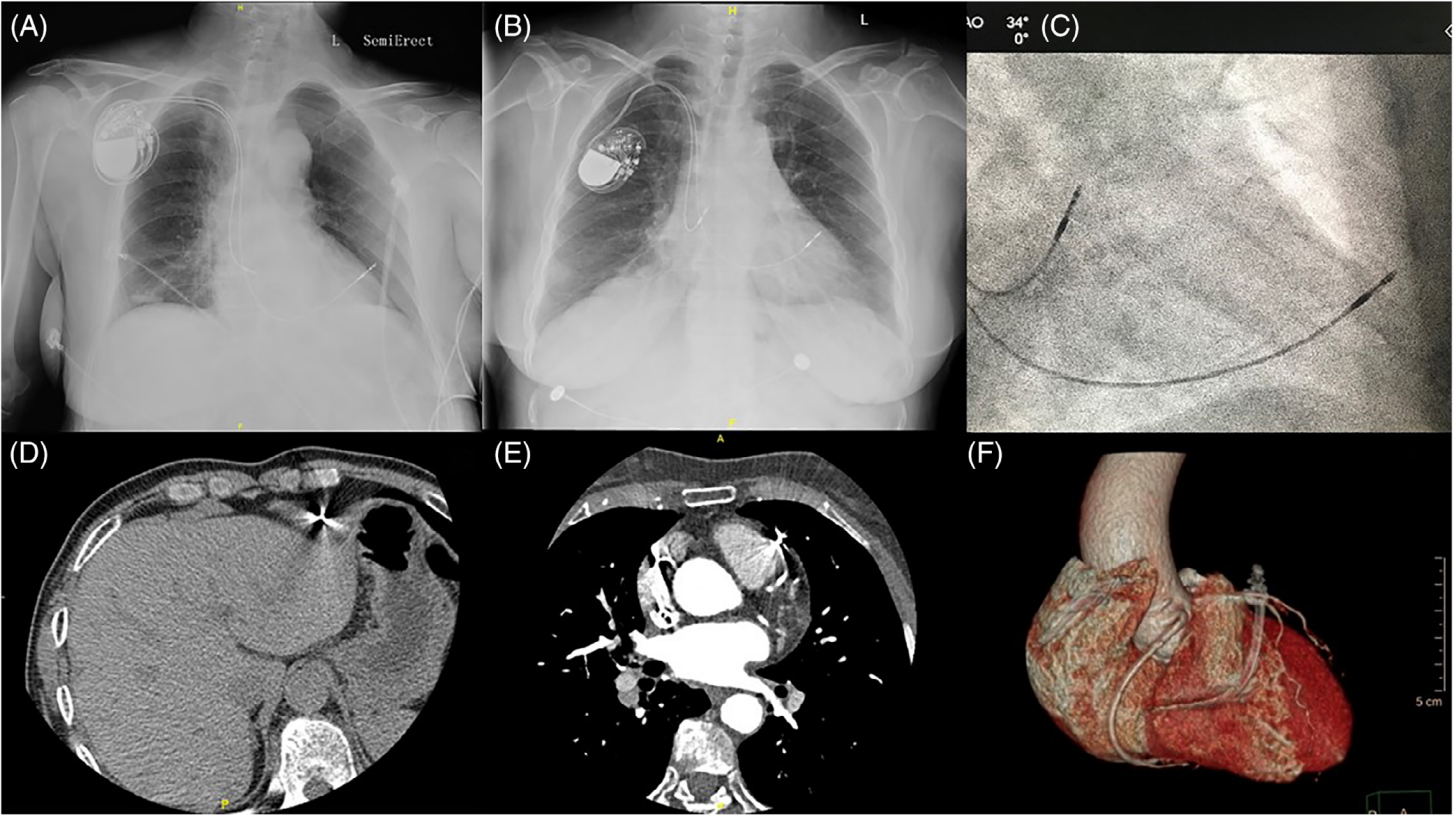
Imaging findings of lead perforation. A, Chest X‐ray demonstrating RV perforation by a PM lead. B, RV lead perforation suspected on AP chest X‐ray. C, RV lead perforation confirmed by fluoroscopy image (same patient as with B) showing lead dislocation outside the border of the heart. D, RV lead shown on chest CT. E and F, lead perforation confirmed by chest CT and corresponding reconstruction (AP view). AP, antero‐posterior; CT, computed tomography; PM, pacemaker; RV, right ventricular
